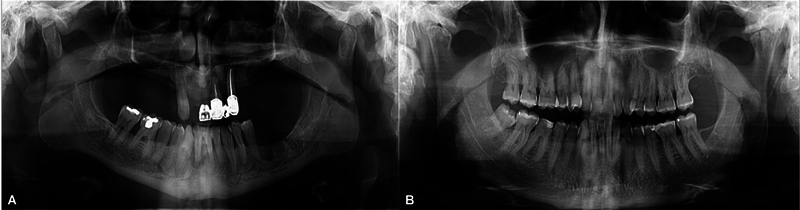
Panoramic radiographs of two 61-year-old male patients: (
